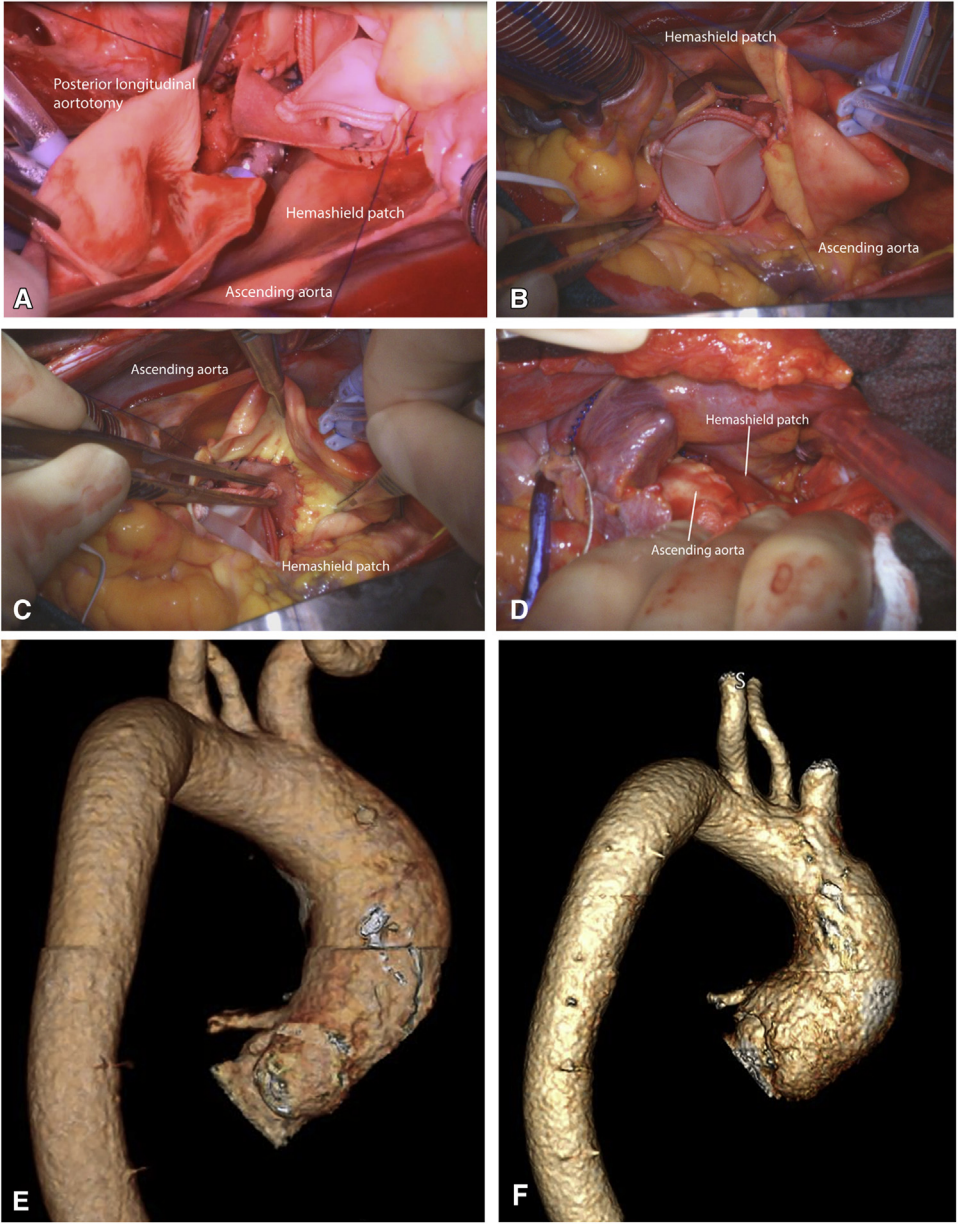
A, The rectangular patch was trimmed into a triangular shape and a longitudinal aortotomy was made to make to incorporate the triangular shape patch into the closure of the complete aortotomy to enlarge the new STJ and proximal ascending aorta. B, The 25 Magna Ease valve (3-valve size upsized); (Edwards Lifesciences) was seated in the enlarged root and triangular patch was partially anastomosed to the longitudinal aortotomy in the ascending aorta. C, The triangular portion (roof) was completely anastomosed to the ascending aorta posteriorly. D, External view of the complete aortotomy closed incorporating the patch at the posterior side of the ascending aorta. E, The preoperative CTA showed the aortic root was 25 × 27 mm, and the STJ was 25 mm. F, The 3-month postoperative CTA showed the aortic root was 35 × 37 mm, and the new STJ was 35 mm. The valve-to-coronary distance was 6 to 7 mm for both coronary ostia.
